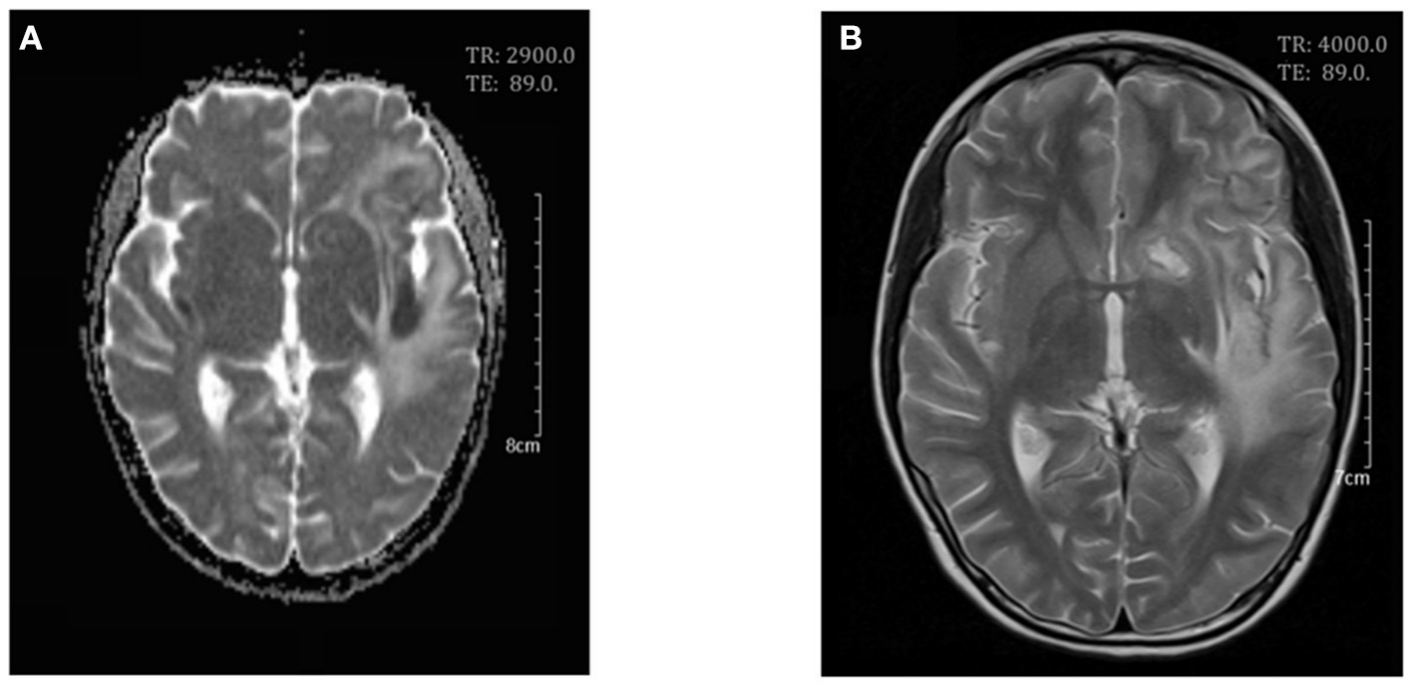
Cerebral magnetic resonance imaging on day 25. Axial T2-weighed scan (A) and apparent diffusion coefficient (ADC) maps (B) showed a decrease in white matter swelling.
